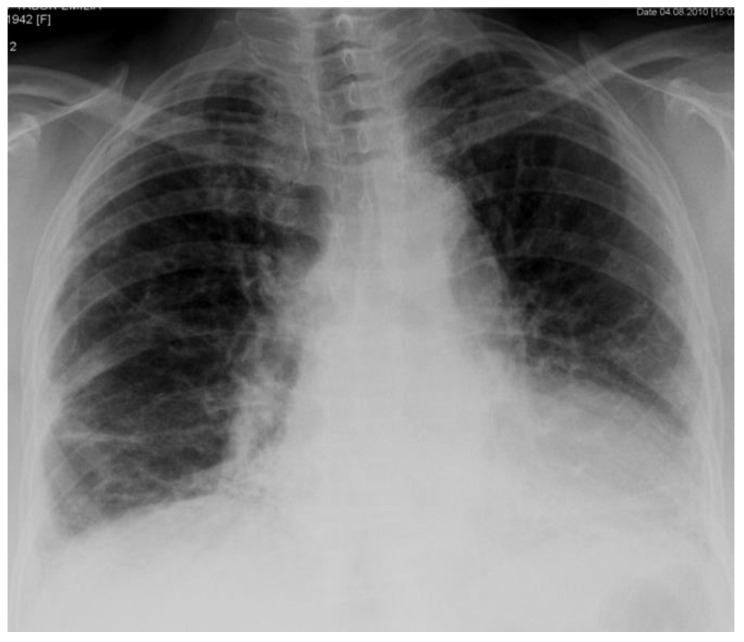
Chest X-ray (2010) shows loss of lung volume and linear opacities in the lung bases and sub-pleural region.
